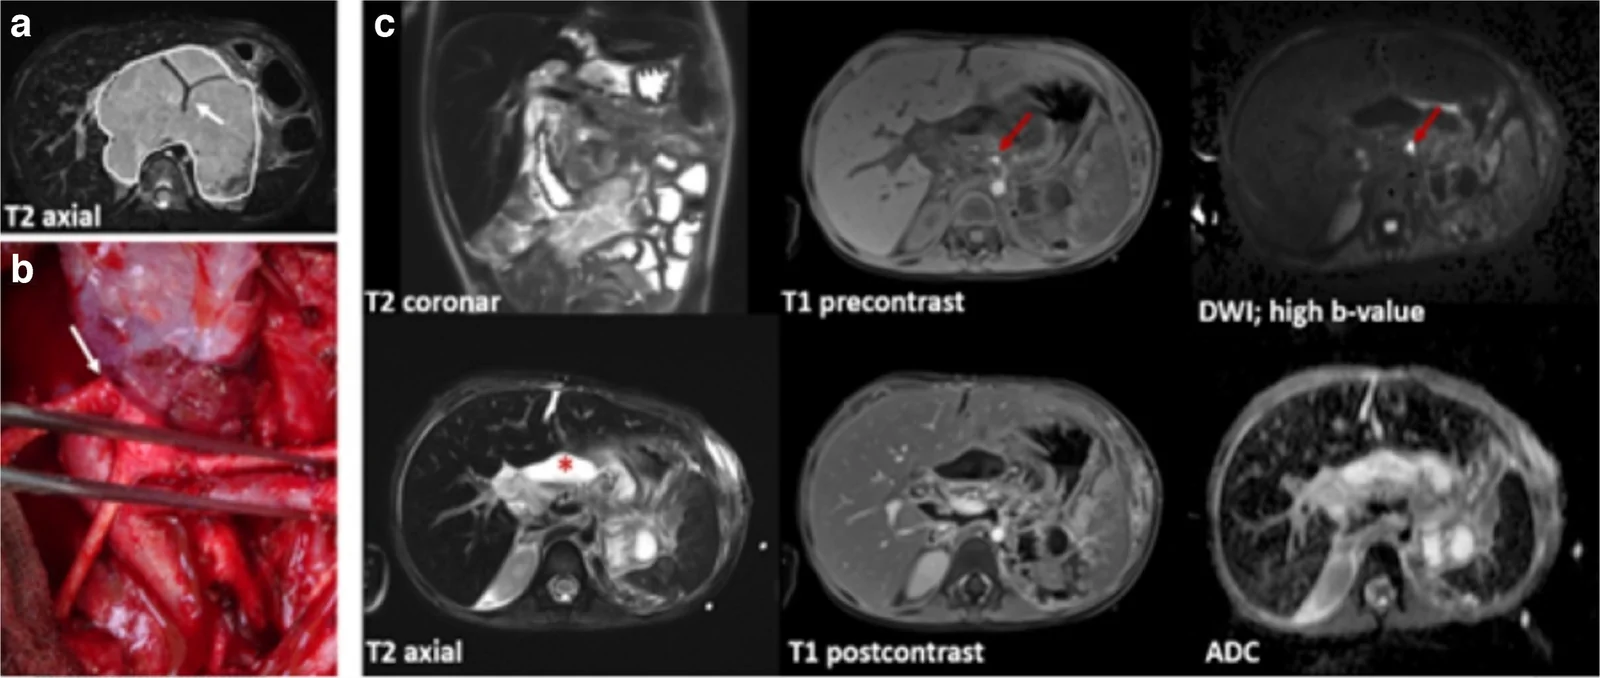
A 2-year-old female patient. a Preoperative magnetic resonance imaging shows a large midline neuroblastoma at the level of the celiac trunk (*white arrow*). b Intraoperative photograph demonstrates macroscopic complete excision (*white arrow*). c Early postoperative magnetic resonance imaging (day 10) shows fluid in the resection area (*red star*) and postoperative hemorrhage without evidence of residual disease (*red arrows*)
